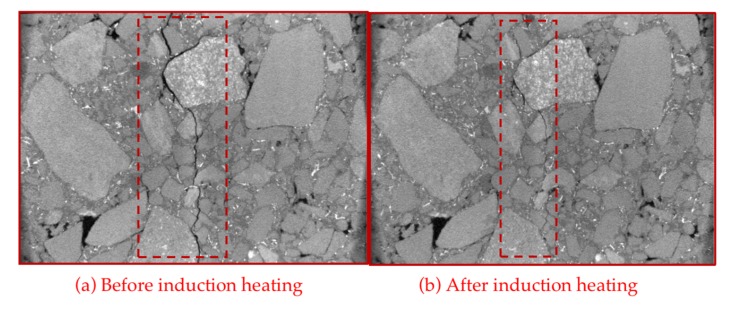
CT scanning figure of SS-6 before and after induction heating.
